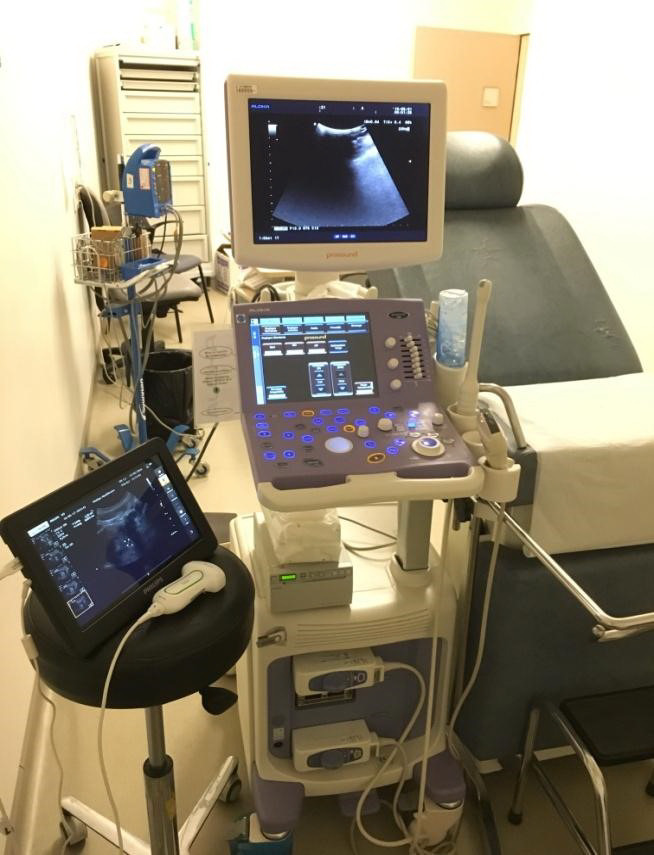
POCUS handheld device (on the left) and comprehensive ultrasound device (on the right) used for IUP detection and gestational age measurement.
